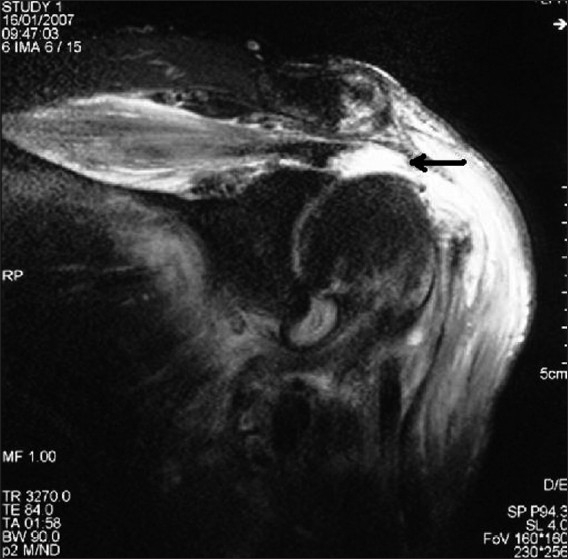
Coronal-oblique T2-weighted magnetic resonance image showing full-thickness rotator cuff tear (arrow), indicated by high signal intensity fluid replacing the insertion of the supraspinatus tendon at the greater tuberosity
